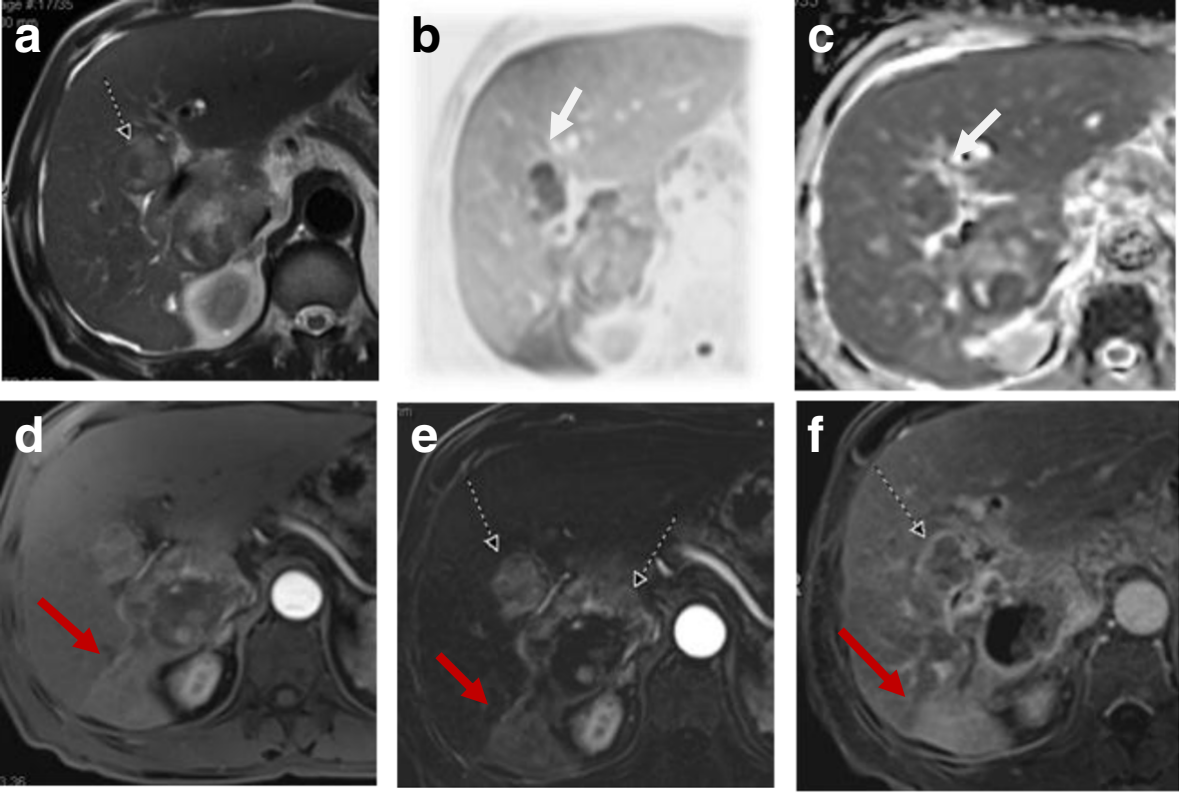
Hepatic focal lesion after TACE. a T2-WI shows two well-defined HFLs of an intermediate to high signal. b, c DWI and ADC show central areas of true restriction for the smaller lesion (arrow) and heterogeneous restriction for the larger one. d Enhanced T1-WI in the arterial phase shows early arterial heterogeneous enhancement. e Subtracted T1-WI arterial phase shows true enhancement of the lesions (dashed arrows). There is a wedge area of enhancement as well (red arrow). f Enhanced T1-WI in the delayed phase shows washout with capsule enhancement (dashed arrow). The wedge enhancement shows delayed T1-WI persistent enhancement (red arrow). Overall post-treatment assessment is LR-TR viable with post-therapy changes
